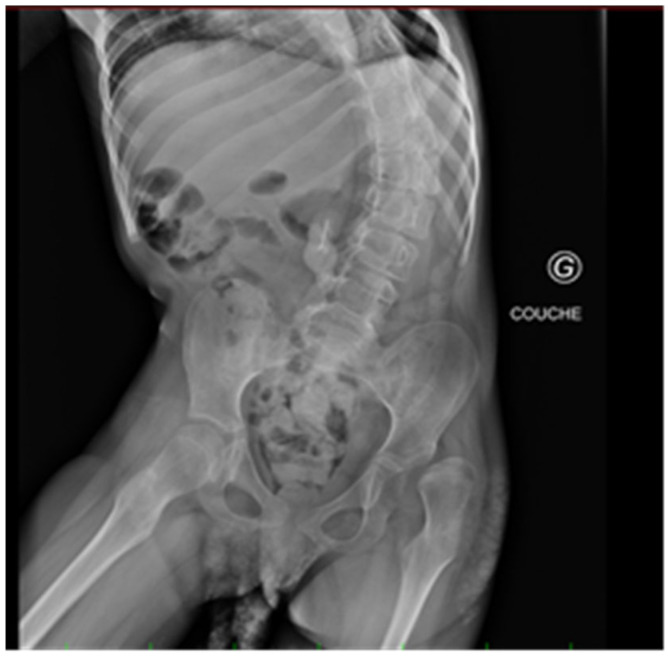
Pelvic X-ray: subluxation of the left hip and coxa valga with thoraco-lumbar scoliosis.
